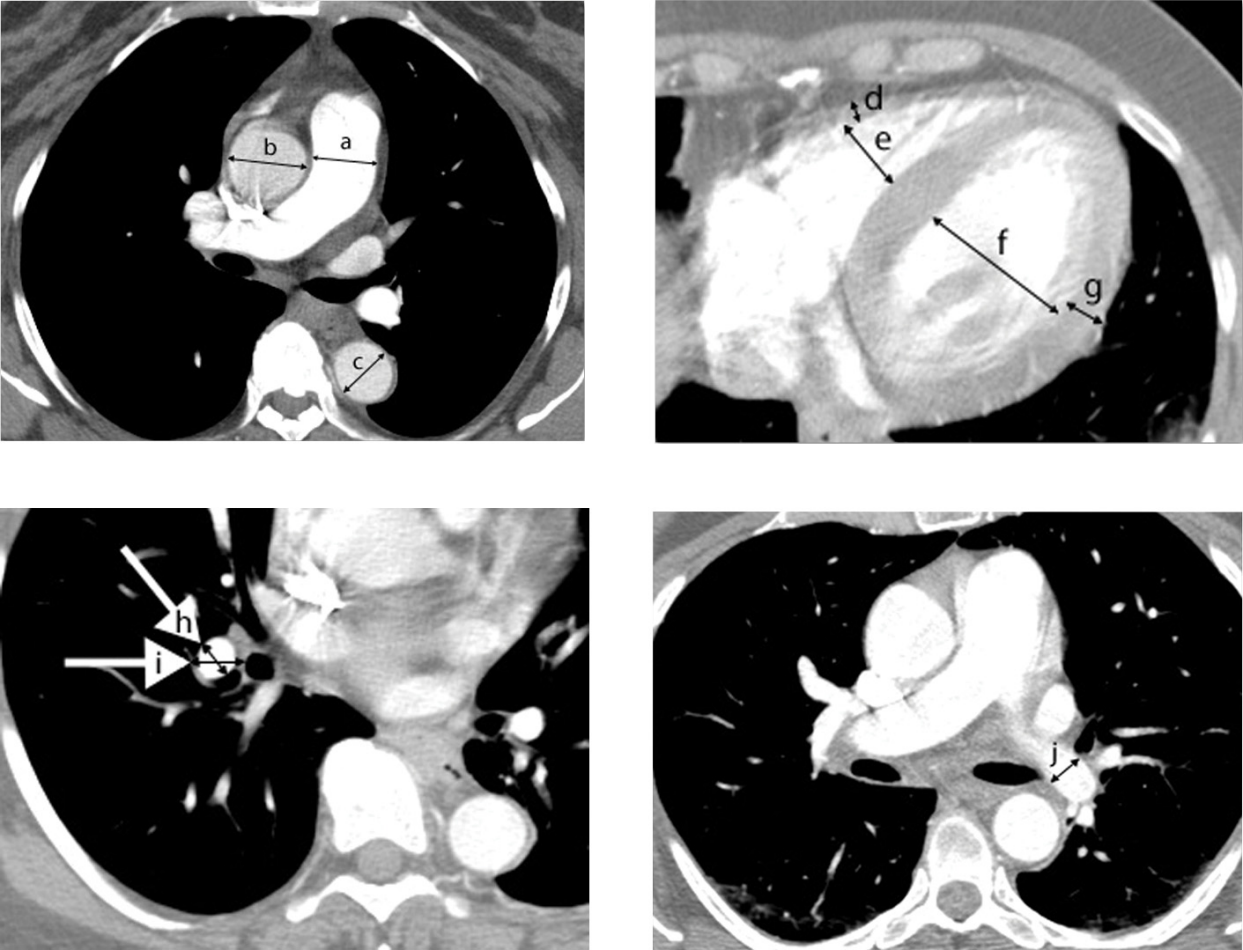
Radiographic measurements. The radiometric measurements used to derive the predictors of PH that were found to be significant in Table 6 included: main PA (a), AA (b), DA (c), RV free wall (d), RV lumen (e), LV lumen (f), LV free wall (g), true RDPA (h), RDPA as would be seen on chest x-ray (i), and true LDPA (j).
